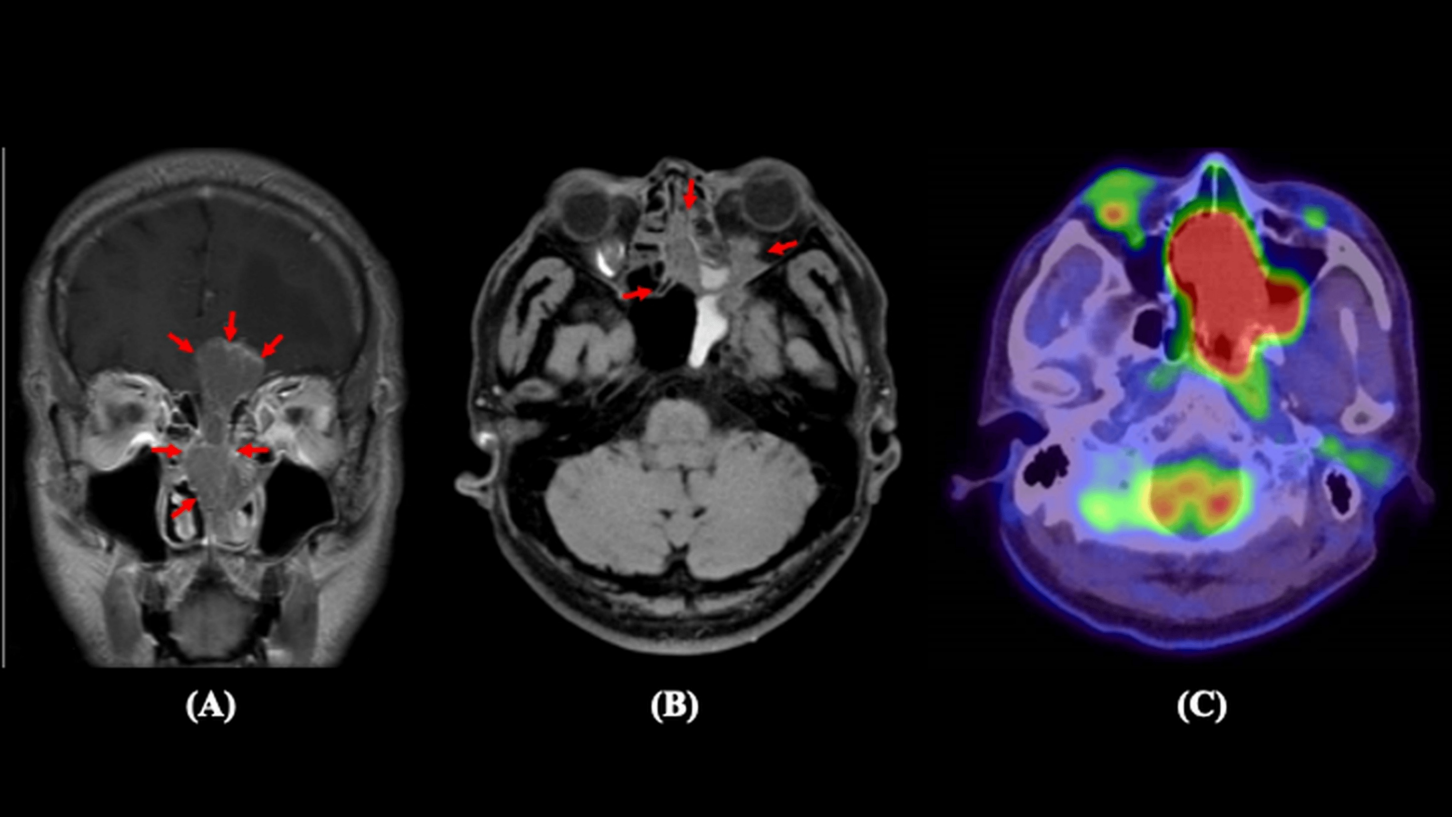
MRI and PET/CT imaging of the sinonasal tumor (A) Coronal and (B) axial T1-weighted MRI images with gadolinium contrast enhancement demonstrate a well-enhancing tumor with extensive invasion into the anterior cranial base, bilateral orbits, and adjacent paranasal sinuses (red arrows).
